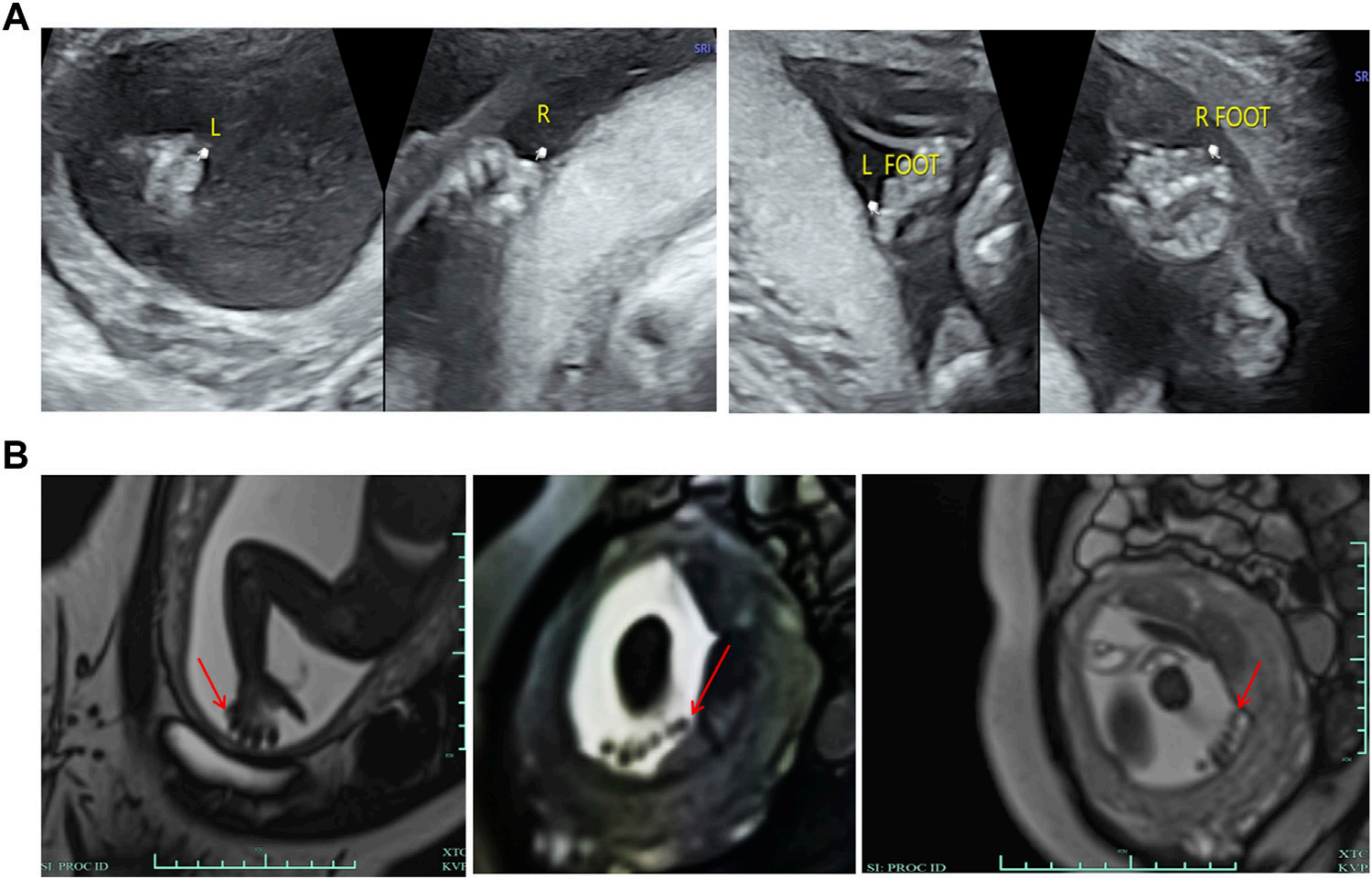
Clinical features of the fetus. (A) Ultrasonography showed that there were echoes of the sixth toe on the lateral side of the little toe of both feet and the lateral side of the little finger of both hands, with a size of about 0.6*0.4 cm, and no obvious bony structure in them. (B) MRI showed a finger-like signal shadow on the outside of the right little finger, while the number of fingers on the left hand was not clear due to the position of the fetus. Six toe-like signal shadows were seen on both feet.
